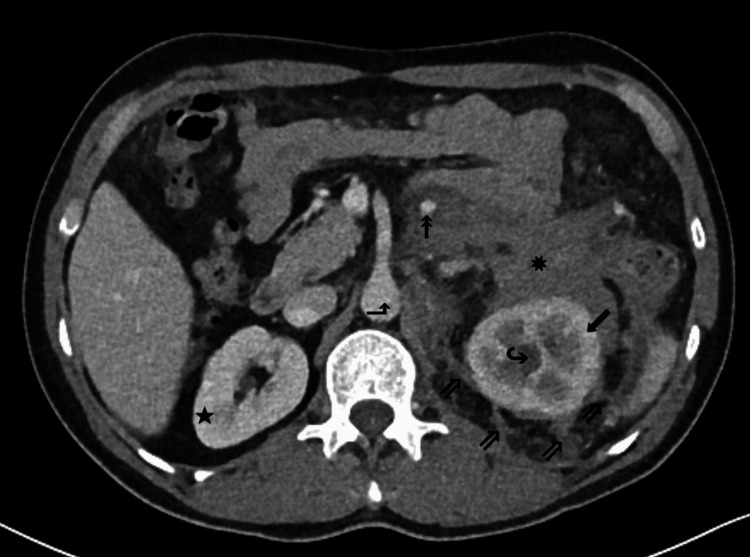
Axial contrast-enhanced abdominal CT at the level of the L1 vertebral body. Left perinephric stranding (⇗) and fascial thickening are present, with a more consolidated appearance of the lesion (✸) anteriorly within the left perirenal space, initially favored to represent a retroperitoneal hematoma. Again noted is delayed enhancement of the left renal cortex (⬋) compared with that of the right kidney (★), with marked left renal pelvocaliectasis (↪), suggestive of a compressive mass effect. At this level, the infiltrative lesion (✸) within the left retroperitoneum encases the inferior mesenteric vein (↟) with relative sparing of the abdominal aorta (⬏).
